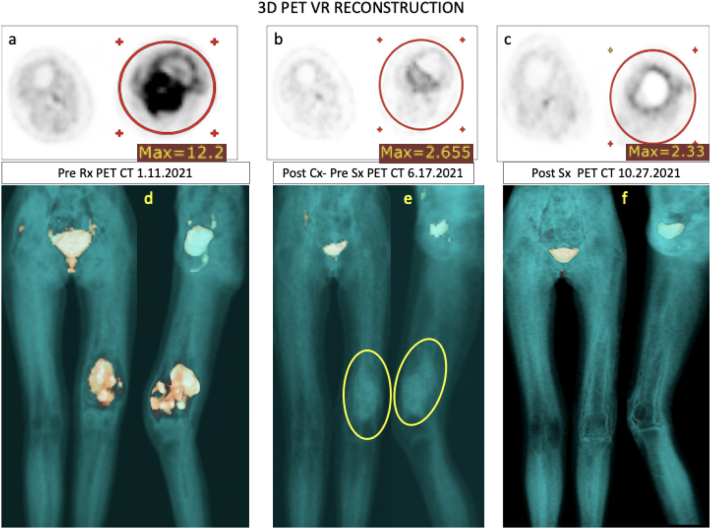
PET-CT scans: Timeline collage of lower extremity unfused PET 3D volume rendered images in anterior and lateral projections with corresponding axial unfused PET images in a & d. pretreatment appearance of an intense focal uptake in the distal left b & e. post treatment appearance of treatment responsive left distal femur with remarkable interval decrease in metabolic uptake of the primary lesion and c & f postsurgical prosthetic replacement of the diseased distal left femur extending across the knee joint devoid of pathological uptake.
